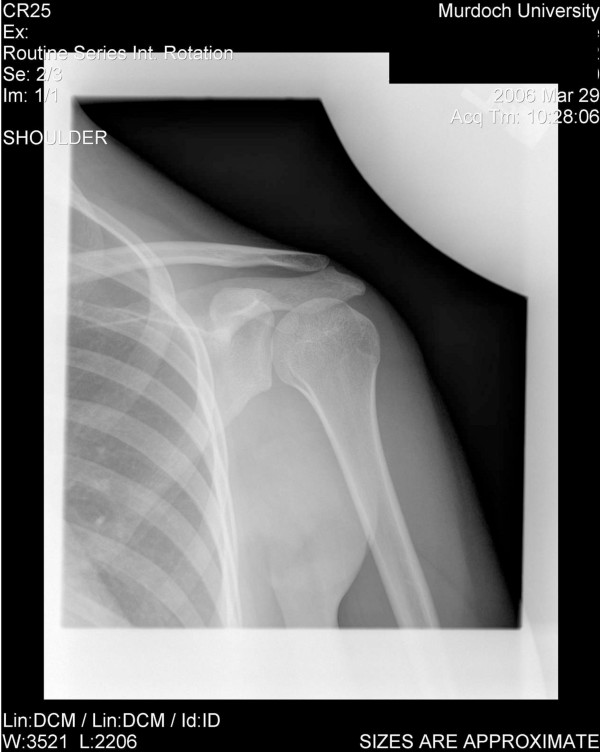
see attached jpeg file named "XRay 2".
